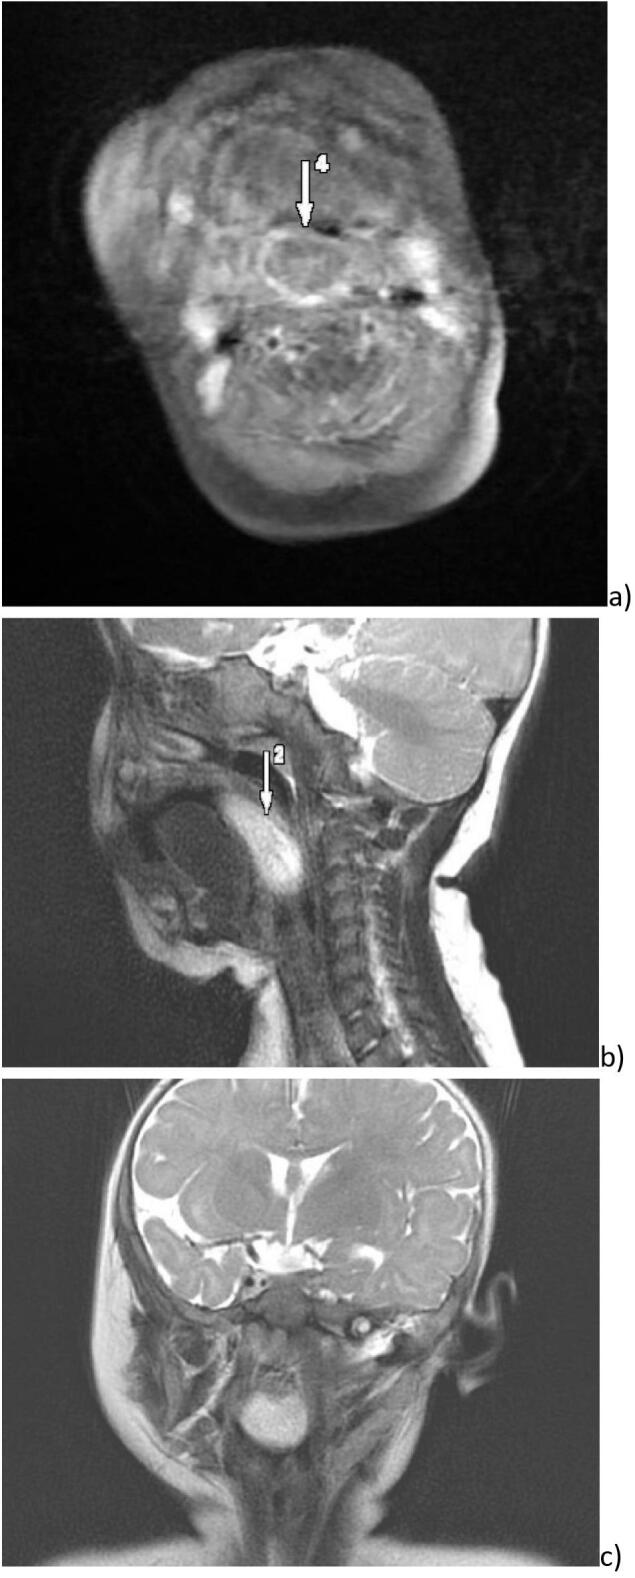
Preoperative MRI in different sections a) axial, b) sagittal and c) coronal showing a well limited tissular lesion in hypersignal in the TWO sequences, this mass is filling the oropharynx.
